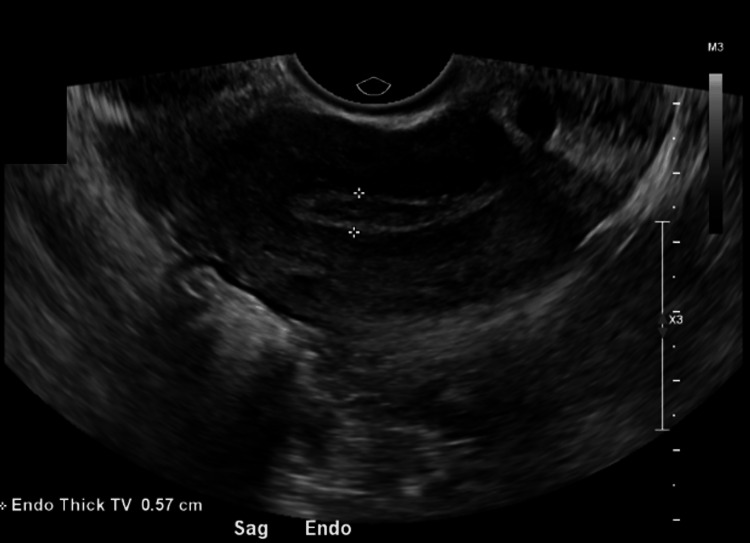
Transvaginal ultrasound on Day 0 showing absence of intrauterine gestation. Initial transvaginal ultrasound obtained on Day 0 demonstrates an empty uterine cavity with an endometrial thickness measuring 0.57 cm. The white arrowheads indicate the endometrial stripe, with no intrauterine gestational sac identified. Findings were concerning for an ectopic implantation, confirmed to be cervical in location.
